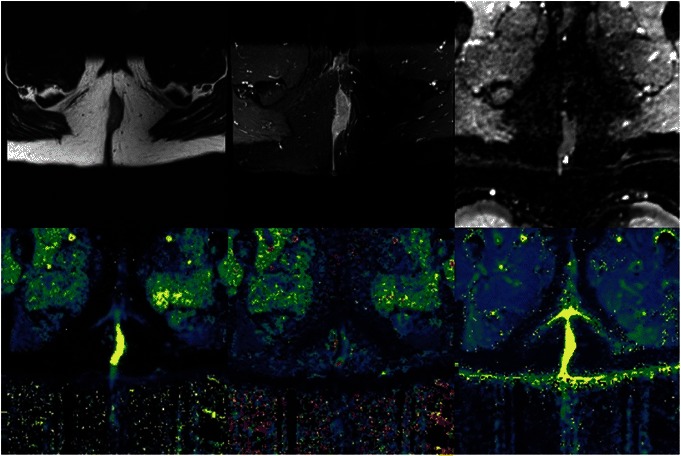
Anal cancer. Morphological MRI (top left and top middle), apparent diffusion coefficient map (top right), dynamic contrast enhanced parametric maps (bottom, from left to right): K_Trans_, K_ep_ and V_e_.
